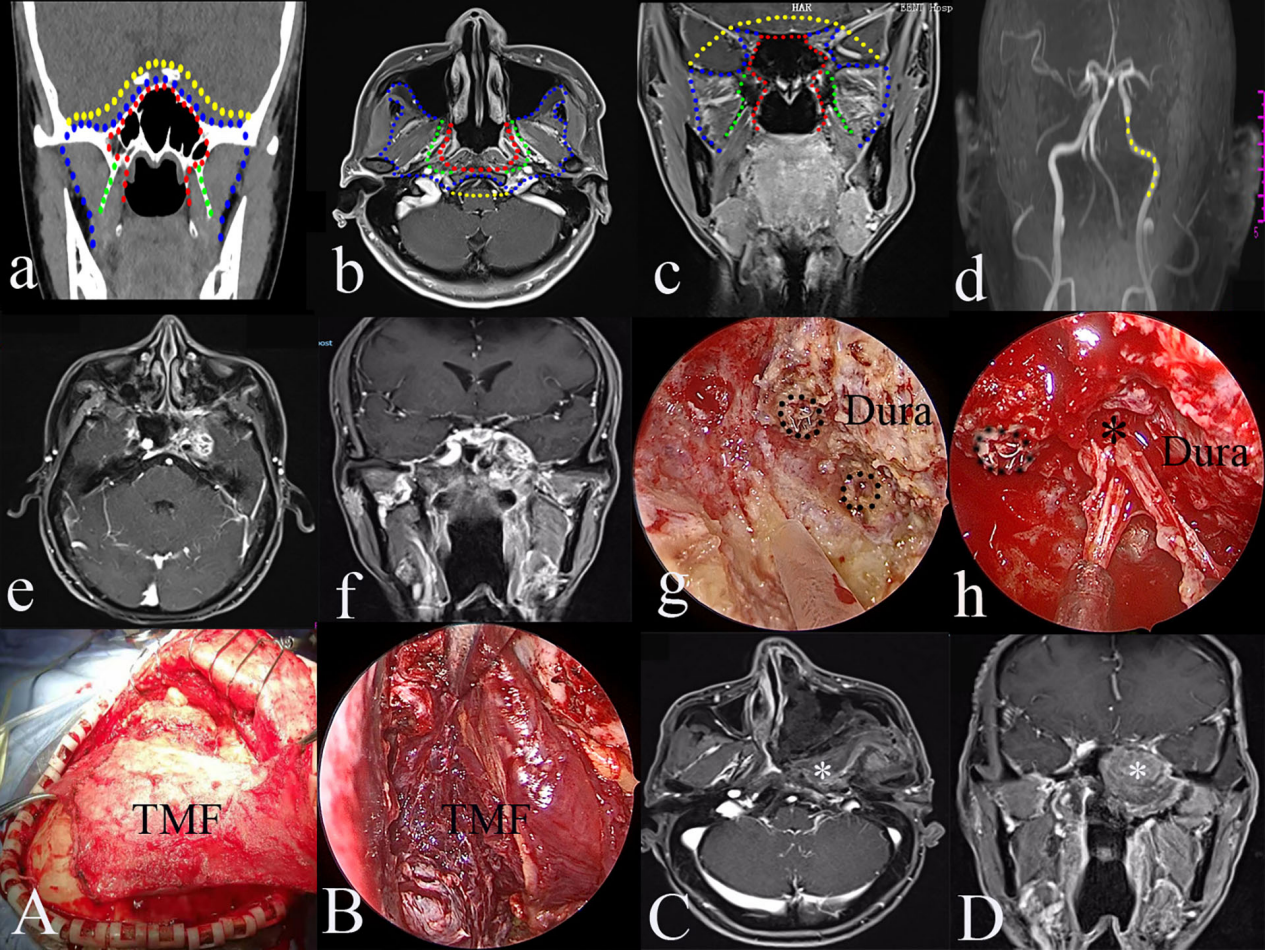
The greatest extent of resection in Type IV transnasal endoscopic nasopharyngectomy (yellow dot line in a–d); yellow dot line indicating the resectable internal carotid artery (ICA) (d); pre-operative magnetic resonance imaging (MRI) of rT4 nasopharyngeal carcinoma (NPC) (e); pre-operative MRA of left occluded ICA (f); resection of the involved ICA (black dot circle) (g); resection of the tumor in the Meckel’s cave (h), black dot circle indicating resected ICA; the reconstruction of the skull defects using TMF (A, B); post-operative MRI indicating the total resection of the tumor (C, D). black *, Meckel’s cave; white *, temporalis muscle flap.
